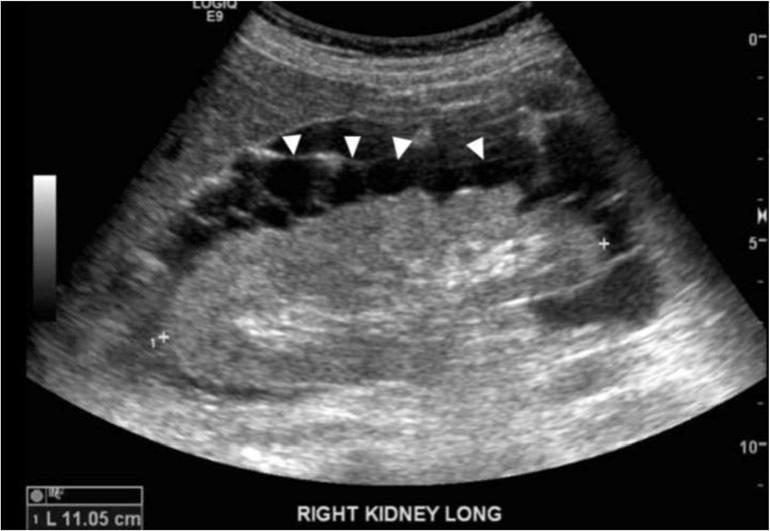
Ultrasound image showing multiple subcapsular cystic lesions (*arrowheads*) bulging into the perinephric space outlining the entire kidney in a patient with lymphangiectasia
